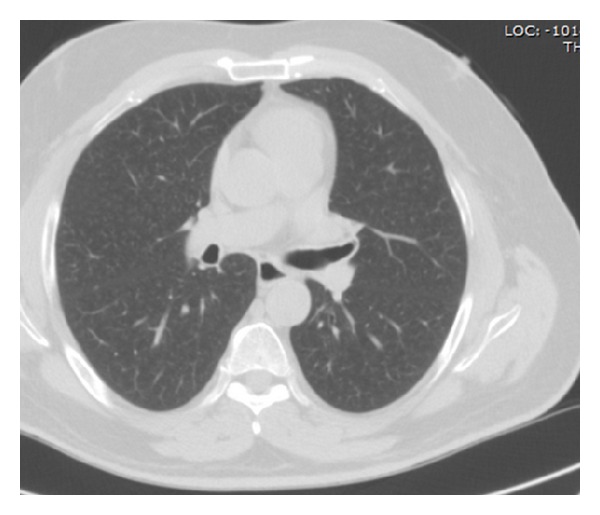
After one-year follow-up, axial noncontrast CT of chest (lung window) showing that the bronchus is still free of masses.
